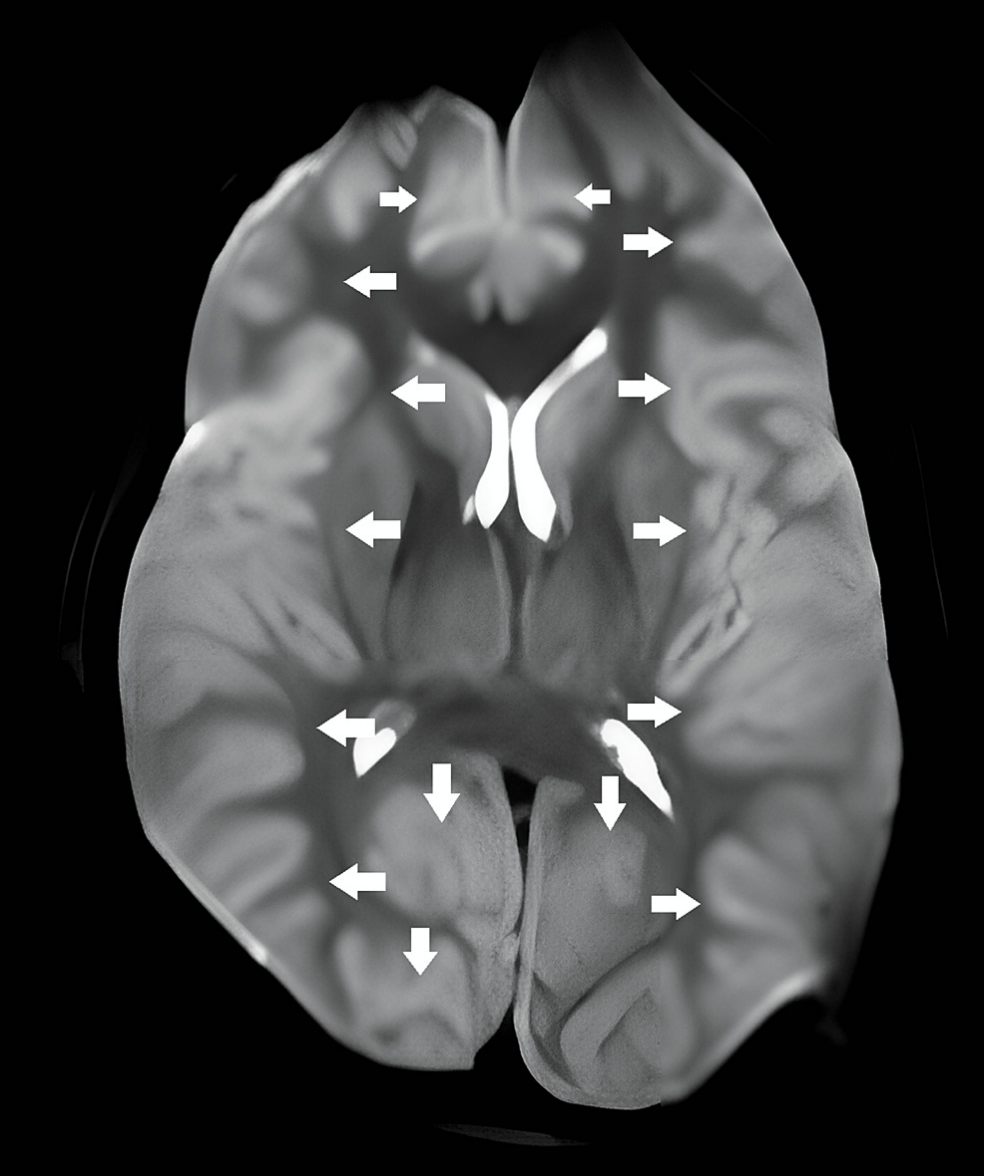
Diffuse hypoxic encephalopathy (white arrows) on brain diffusion MRI on the third postoperative day.
